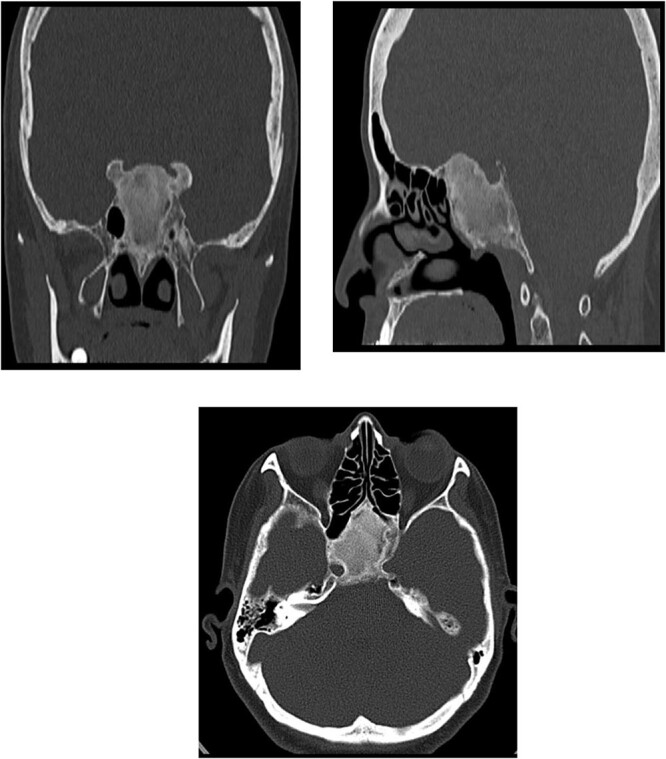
CT of the paranasal sinuses showing expansion of the sphenoid bone demonstrating diffuse ground-glass matrix and sclerosis obliterating the sphenoid sinus cavity.
